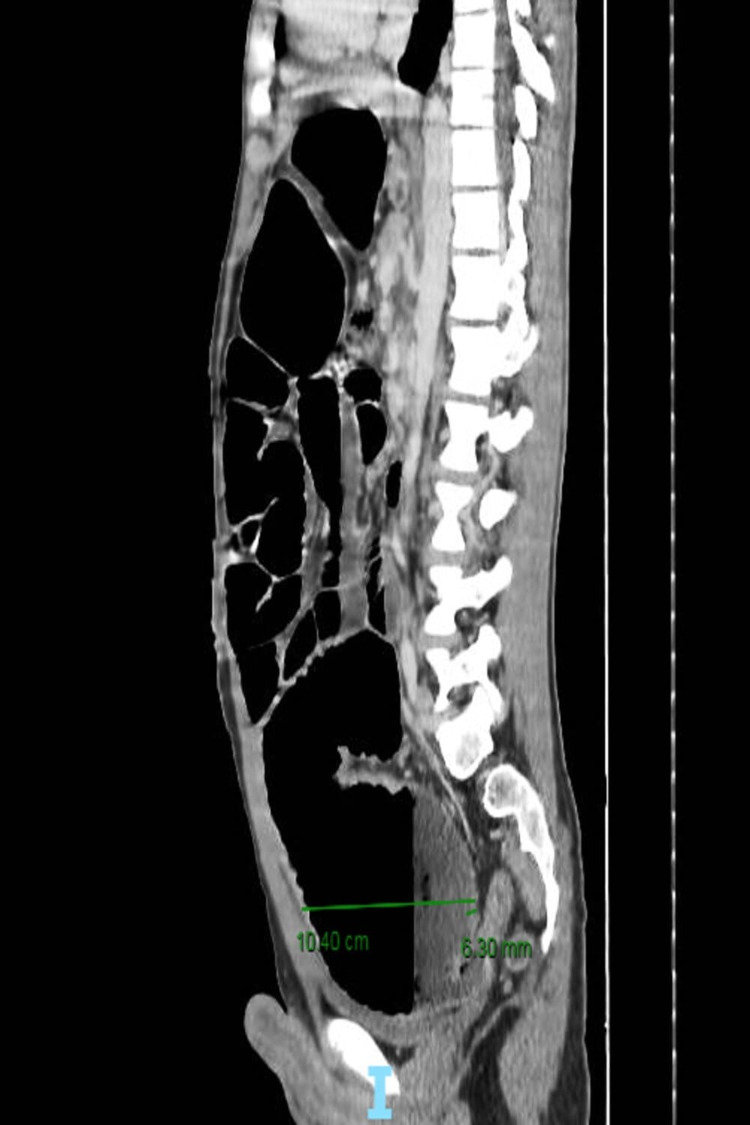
CT scan showing dilated colon and intestine in the sagittal section.
